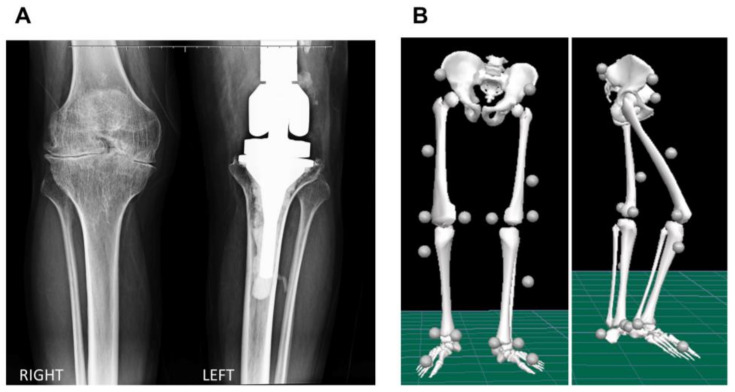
(A) Standing anterior–posterior radiographic view of the right and left knees, and (B) frontal and sagittal view of the biomechanical model displaying the marker set convention adopted in the study.
